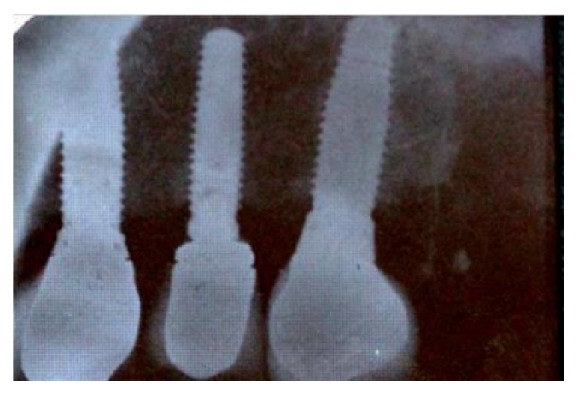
Intraoral radiograph at the 1-year follow-up.
